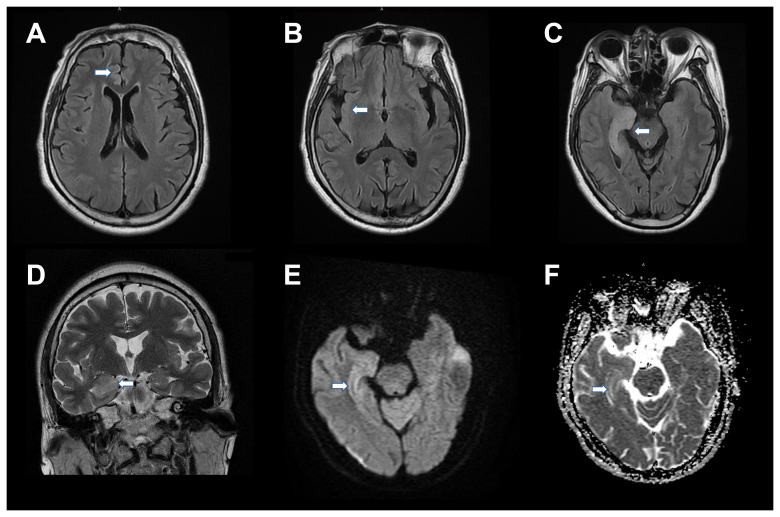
Admission magnetic resonance imaging showing right mesial temporal and multifocal cortical hyperintensities. Axial fluid-attenuated inversion recovery images demonstrate hyperintense signal in (A) the cingulate gyri and (B) the insular cortices, with right-sided predominance (arrows). (C) Axial fluid-attenuated inversion recovery image at the level of the mesial temporal lobe and (D) coronal T2-weighted image show right hippocampal and mesial temporal lobe hyperintensity (arrows). (E) Diffusion-weighted imaging shows apparent high signal in the right hippocampus (arrow); (F) the corresponding apparent diffusion coefficient map demonstrates elevated signal, consistent with T2 shine-through rather than true restricted diffusion (arrow).
